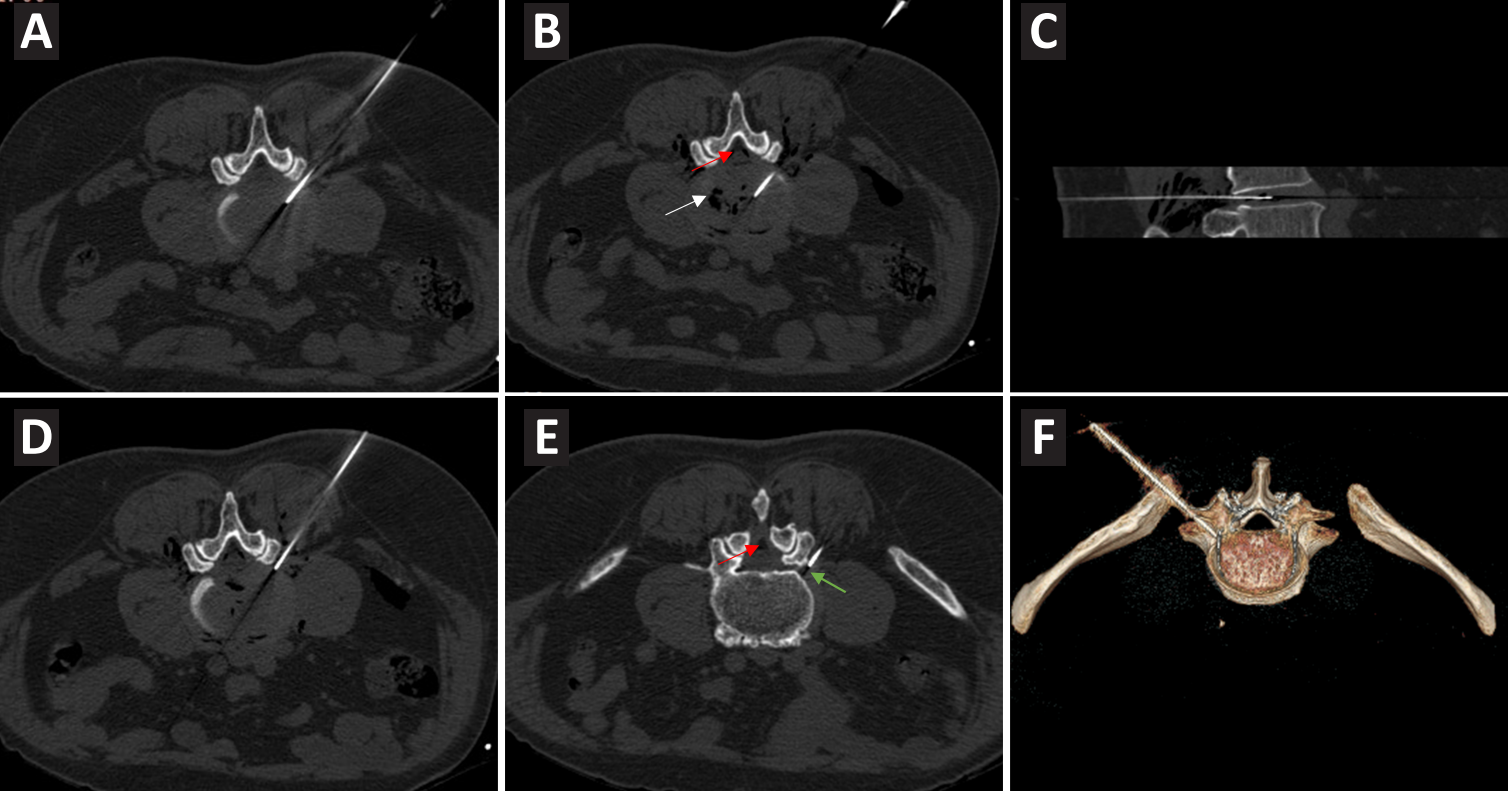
CT-guided injection of medical oxygen–ozone for the treatment of lumbar disc herniation combined with spinal canal stenosis. (A) The needle tip of the syringe is positioned at the posterior center of the affected intervertebral disc. (B) Injection of oxygen–ozone into the center of the affected intervertebral disc. (C) Sagittal view showing the needle tip positioned within the affected intervertebral disc. (D) The needle tip is withdrawn to the area near the intervertebral foramen. (E) Injection of oxygen–ozone near the intervertebral foramen. (F) Three-dimensional reconstruction image during the injection process. White arrow indicates visible gas; red arrow indicates gas within the spinal canal; green arrow indicates visible gas. CT: Computed tomography; LDH: lumbar disc herniation; LSS: lumbar spinal stenosis.
